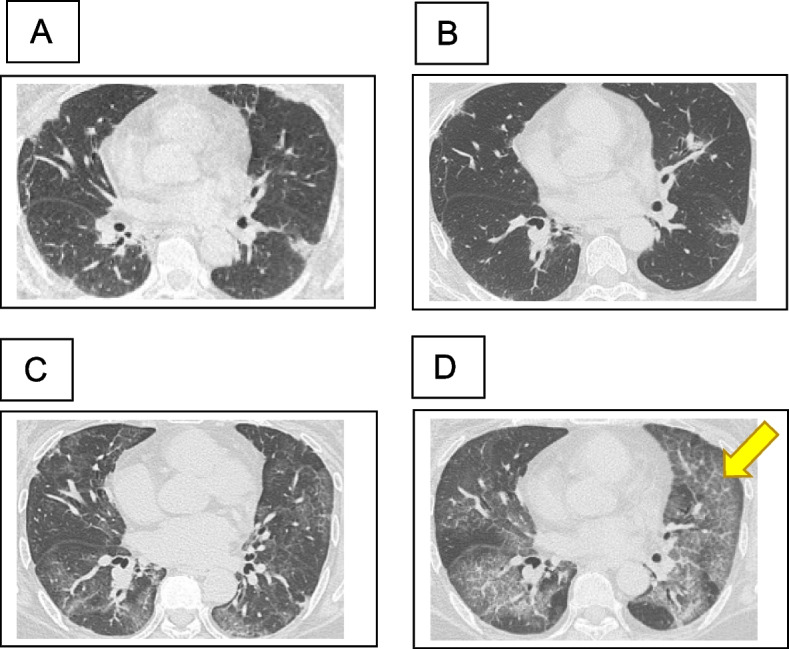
Course of findings of interstitial pneumonia on chest computed tomography (CT). A At the diagnosis of dermatomyositis, B 2 months after initiation of treatment, C 8 months after initiation of treatment, and D 14 months after initiation of treatment (before bronchoscopy). On initial admission (A), interstitial shadows were observed just below the pleura in both lungs. At discharge 2 months later, the interstitial shadows temporarily improved (B). However, 8 months later (C), new ground-glass shadows appeared in both lungs. Fourteen months later (D), the ground-glass shadows were further aggravated, as indicated by the arrow
